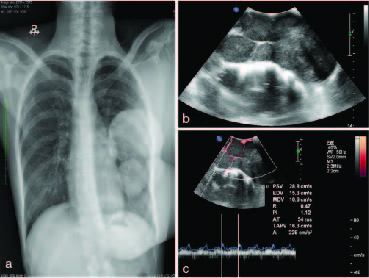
(a) Chest radiograph showing a large multilobulated soft tissue density lesion involving the left breast with normal bilateral lung fields and bony thoracic cage. (b) Ultrasound of the left breast revealing a circumscribed multilobulated solid heteroechoic lesion with internal vascularity on (c) colour Doppler. AI, average intensity; AT, average time; EDV, end-diastolic velocity; MDV, mean diastolic velocity; PI, pulsatility index; PSV, peak systolic velocity; PW, pulsed wave; RI, resistive index; SV, stroke volume; TAPV, time-averaged peak velocity.
